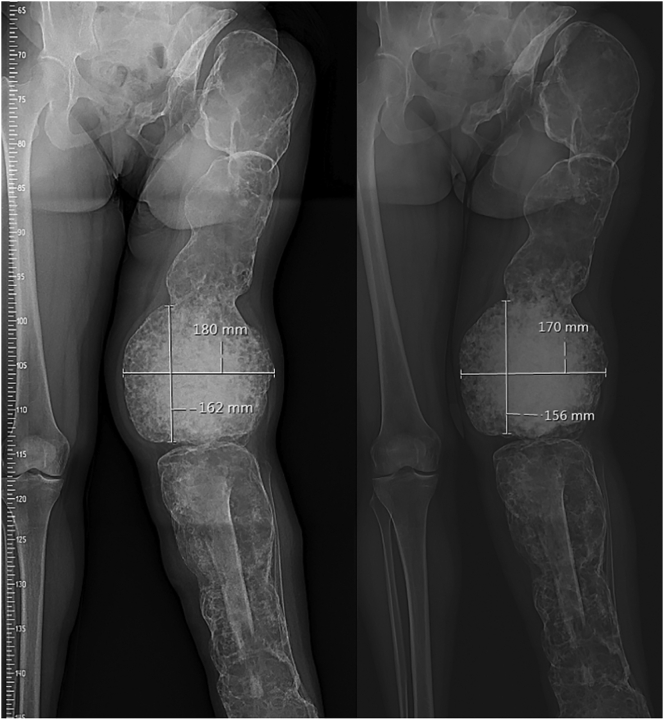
Regression of the lesions in the distal femur before (left) and after 3 years of dmab therapy (right) on plain X-ray of case 1. In the tibia, the bone allograft is visible which was transplanted during previous surgery.
